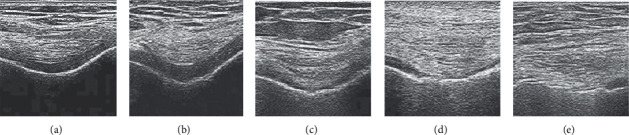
The ultrasound imaging manifestations of different stages in the control group. (a) Normal. (b) Stage I. (c) Stage II. (d) Stage III. (e) Stage IV.
